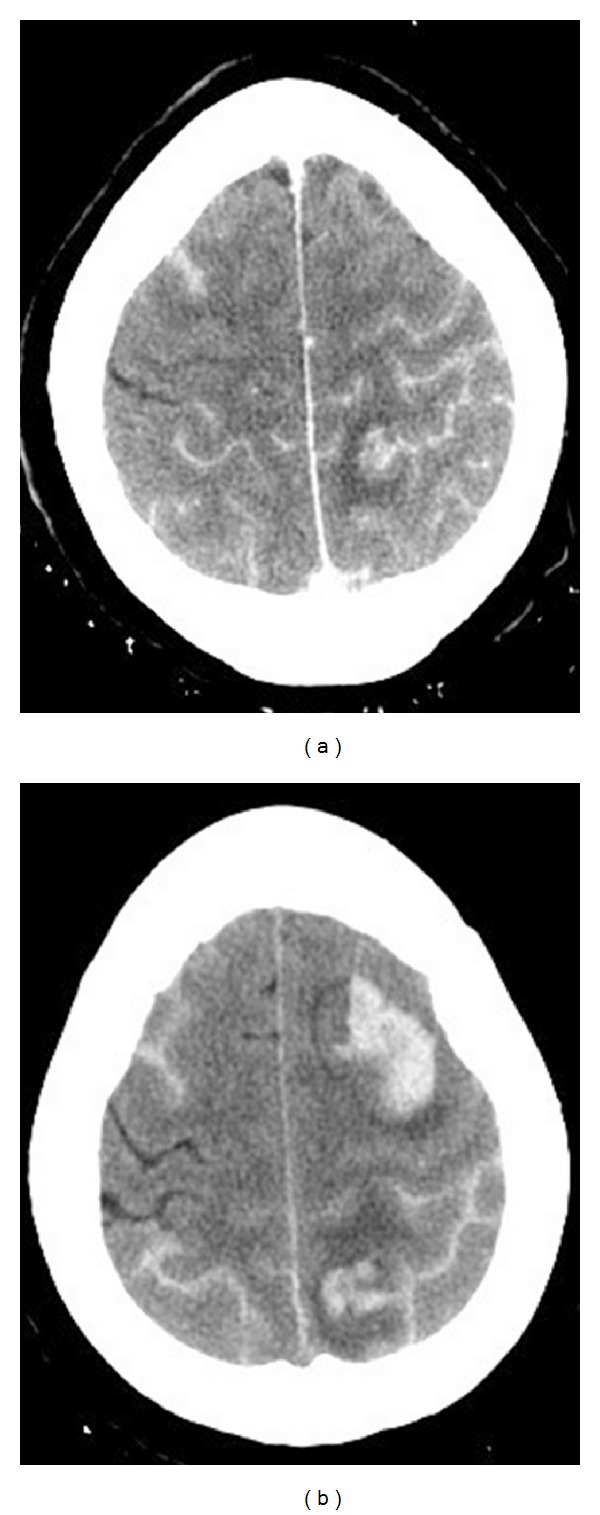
Initial cranial CT demonstrated convexity subarachnoid hemorrhage and a 1.5 cm left superior parietal acute intraparenchymal hemorrhage (a). Three days later, the patient developed acute right hemiplegia. Repeat CT demonstrating a new 3.3 × 1.7 cm left frontal intraparenchymal hemorrhage (b).
